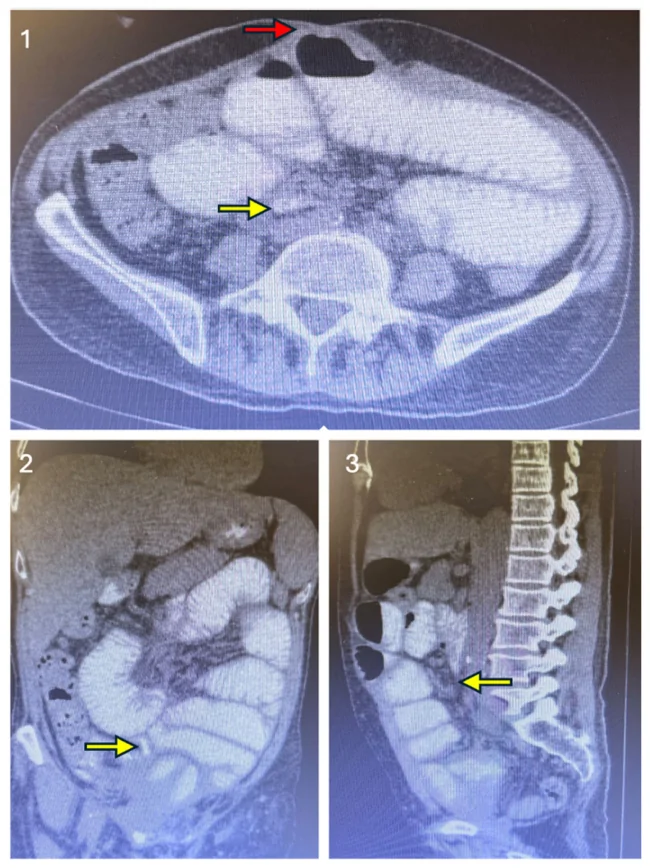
Preoperative computed tomography in a patient with a prior cystectomy for bladder tumor (Patient 10). (1) Axial slice: the red arrow indicates the planned trephine/stoma site, and the yellow arrow indicates the level of obstruction. (2) Coronal reformatted image: yellow arrow indicates the level of obstruction. (3) Sagittal reformatted image: yellow arrow indicates the level of obstruction. The obstructed segment was located approximately 30 cm proximal to the planned target segment and, based on CT measurement, approximately 200 cm distal to the ligament of Treitz; this localization supported the decision for loop ileostomy.
